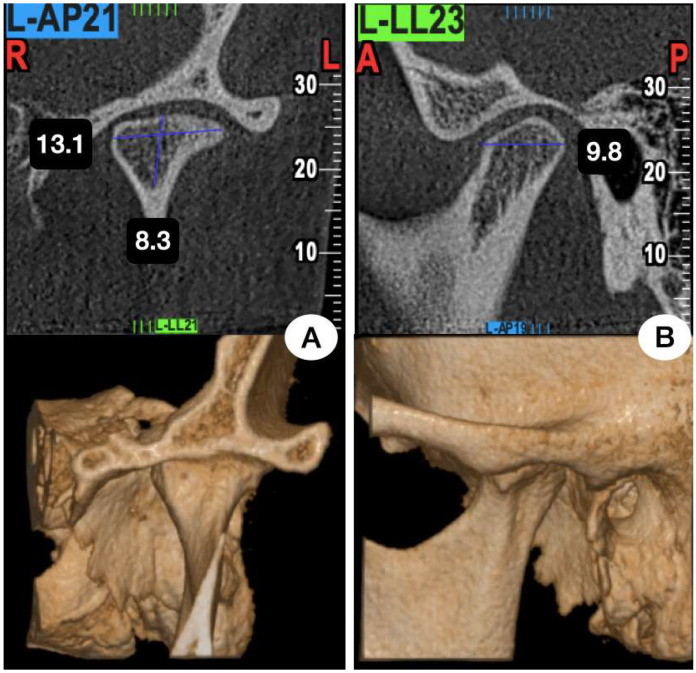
CBCT frontal (A) and sagittal (B) sections of TMJ with degenerative process and morphological change of the condyle.
